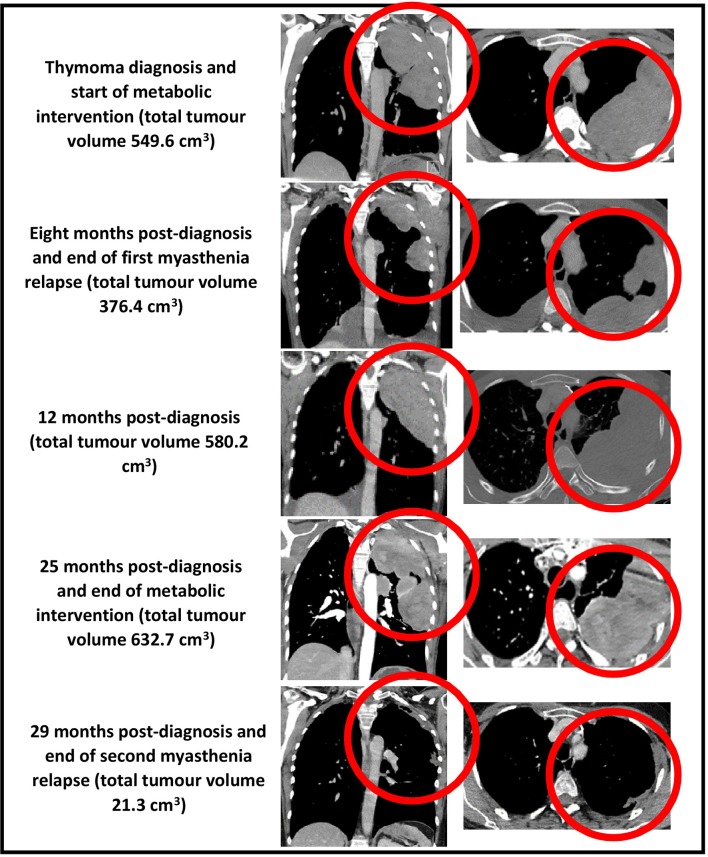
CT chest (coronal and axial views, bulk of tumor circled in red) showing total tumor volumes at diagnosis, 8, 12, 25, and 29 months post-diagnosis.
